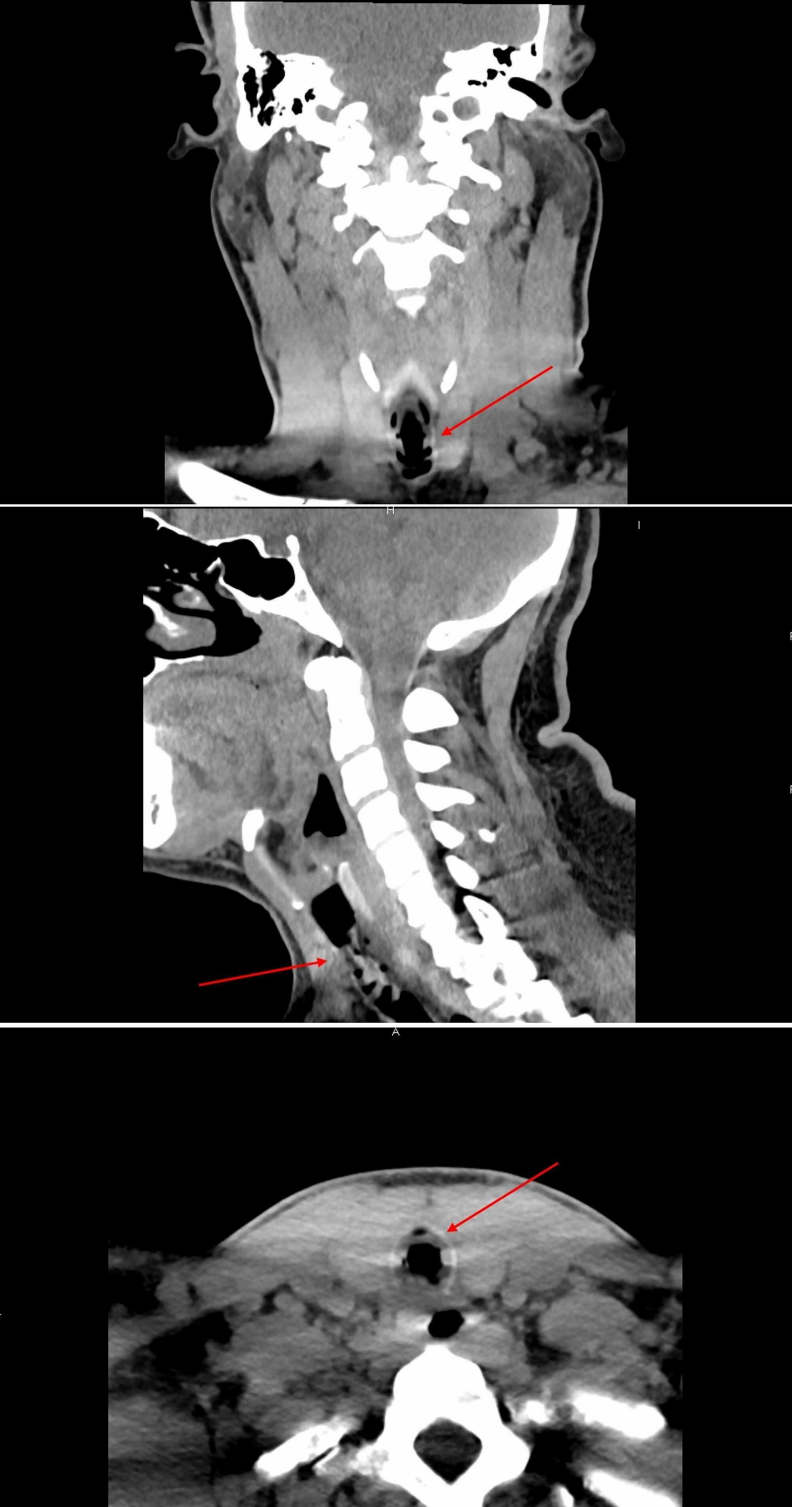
Soft Tissue Neck CT scan showing prominent airway narrowing throughout the nasopharynx and oropharynx based on the prominence of the lymphoid soft tissues and markedly abnormal trachea below the cricoid cartilage with the presence of stenosis (arrows)
